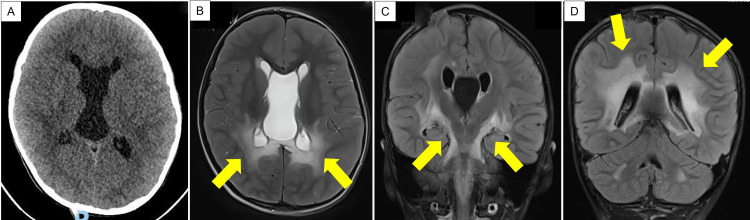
Representative neuroimaging of the patient at the time of initial presentation (A) Representative non-contrasted CT axial image depicting enlarged cavum septum pellucidum et cavum vergi with hydrocephalus. (B) Representative MRI axial image delineating a cavum vergae cyst causing hydrocephalus in T2-weighted sequence. Symmetrical areas of T2-weighted signal are noted in the periventricular white matter of bilateral parieto-occipital regions (yellow arrows). These latter findings were initially interpreted as transependymal CSF seepage due to obstructive hydrocephalus. (C and D) Representative MRI images in FLAIR sequence in coronal views. Both images highlight the extent of the signal changes in the periventricular white matter (D), especially involving lateral aspects of both thalami, cerebral peduncles, midbrain, and pons (C) (yellow arrows point to pertinent areas of MRI signal changes in both images). FLAIR: fluid-attenuated inversion recovery
